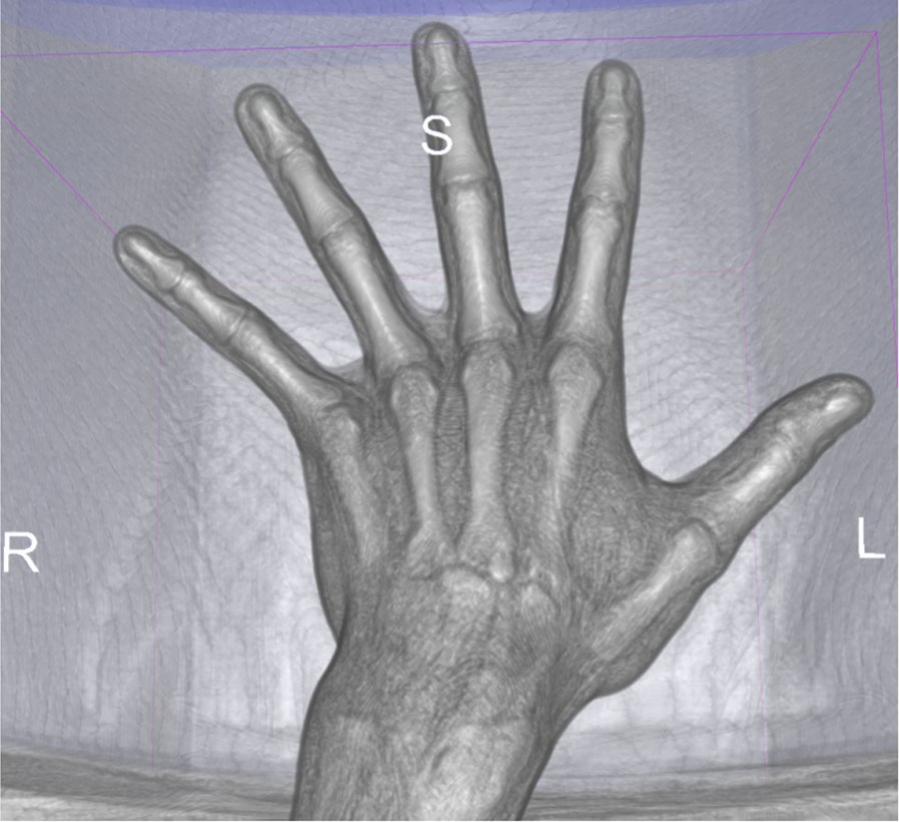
Visualization of captured 3D data from CT in 3D Slicer software. Using opaque tissues volume rendering to be able to see the bones because of precise setting of the region of interest
